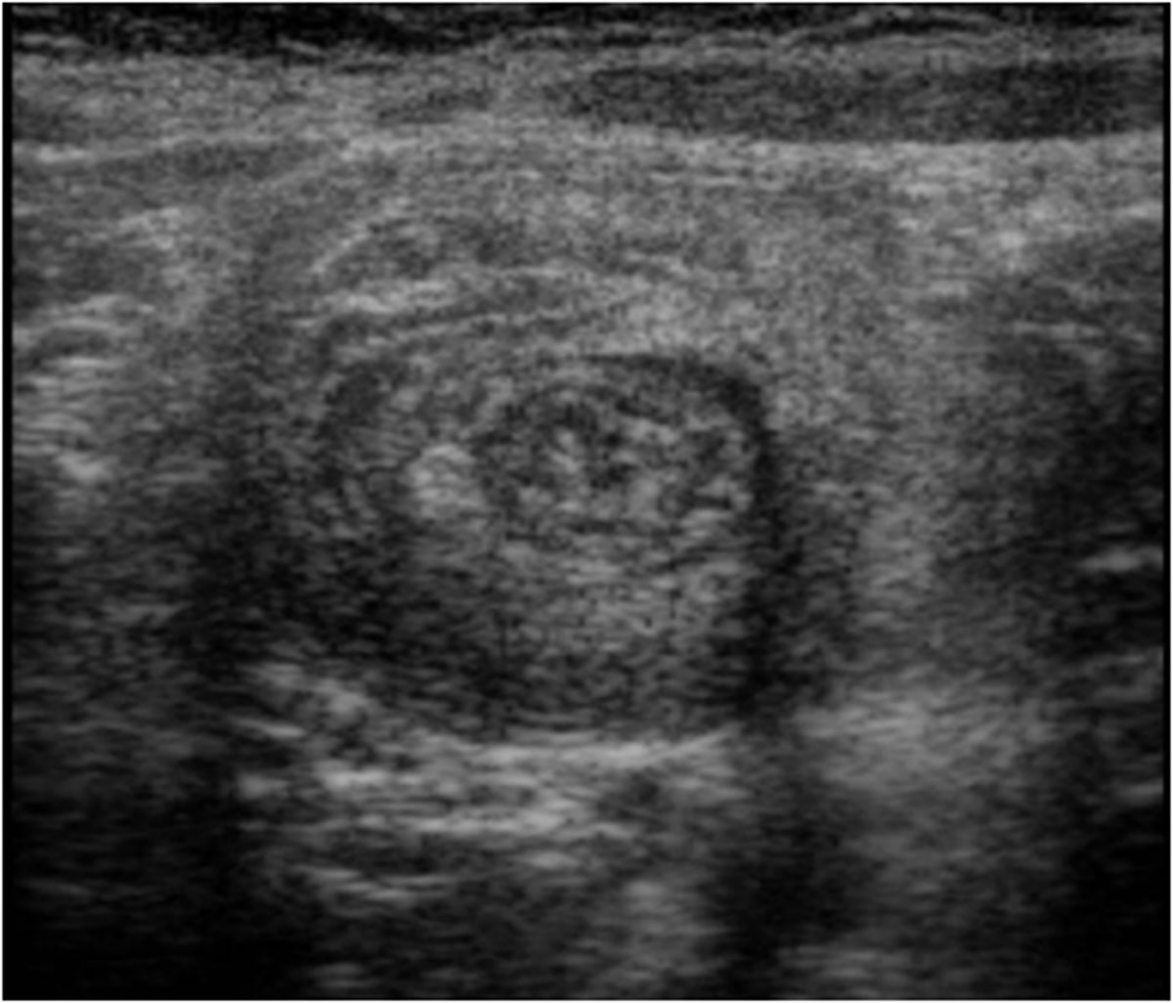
Ultrasound features of intussusceptions. Us obtained near the apex shows multiple concentric rings with a hypo-echoic ring surrounding a hyper-echoic ring, which surrounds another hypo echoic ring.
